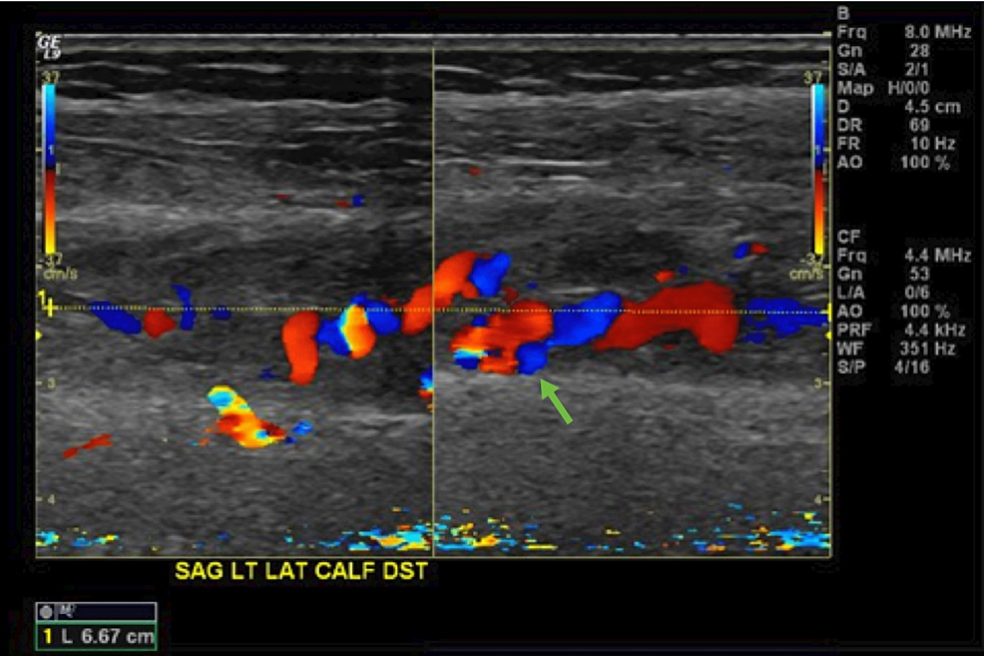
Duplex ultrasound of the left lower-extremity arteriovenous malformation demonstrating an abnormal intramuscular vasculature (arrow).
